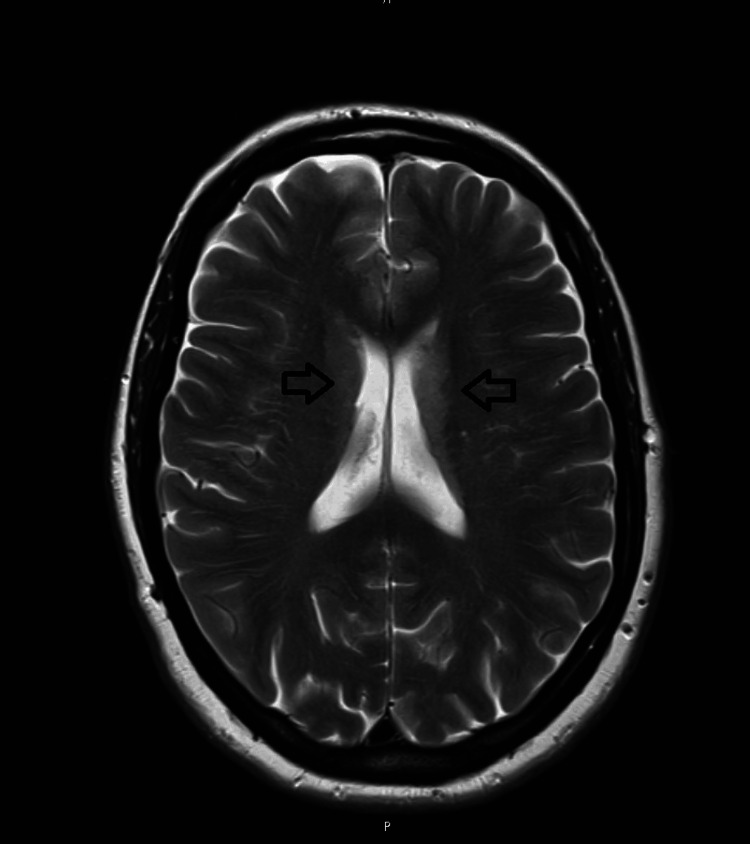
Magnetic resonance imaging (MRI) image of the brain Abnormal diffusion restriction in the basal ganglia and bilateral thalami as described is concerning; differential includes Creutzfeldt–Jakob disease (CJD), recent seizure activity, and previous hypoxic/metabolic encephalopathy.
